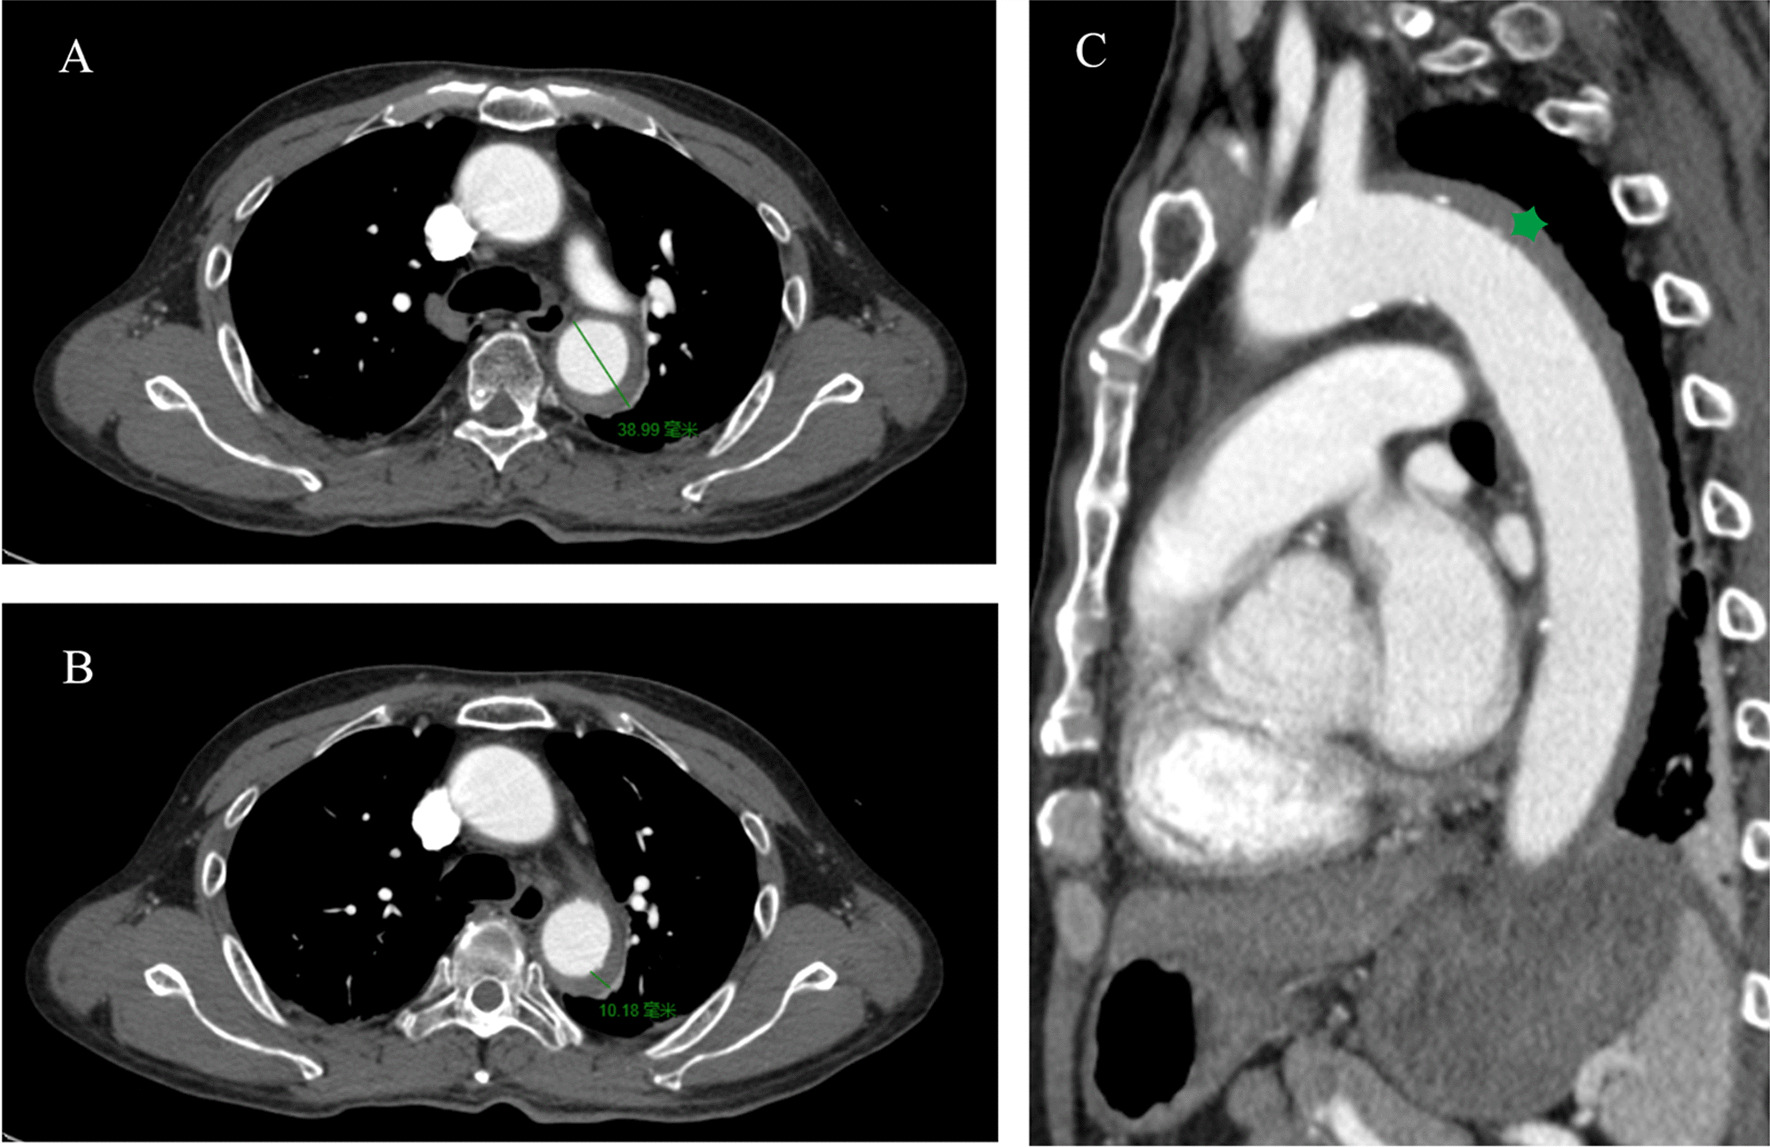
The urgent contrast-enhanced computed tomography image on admission shows intramural hematoma of the descending aorta. A The section of the maximal aortic diameter (line). B The section of maximal hematoma thickness (line). C Sagittal contrast-enhanced computed tomography shows intramural hematoma of the descending aorta (star)
